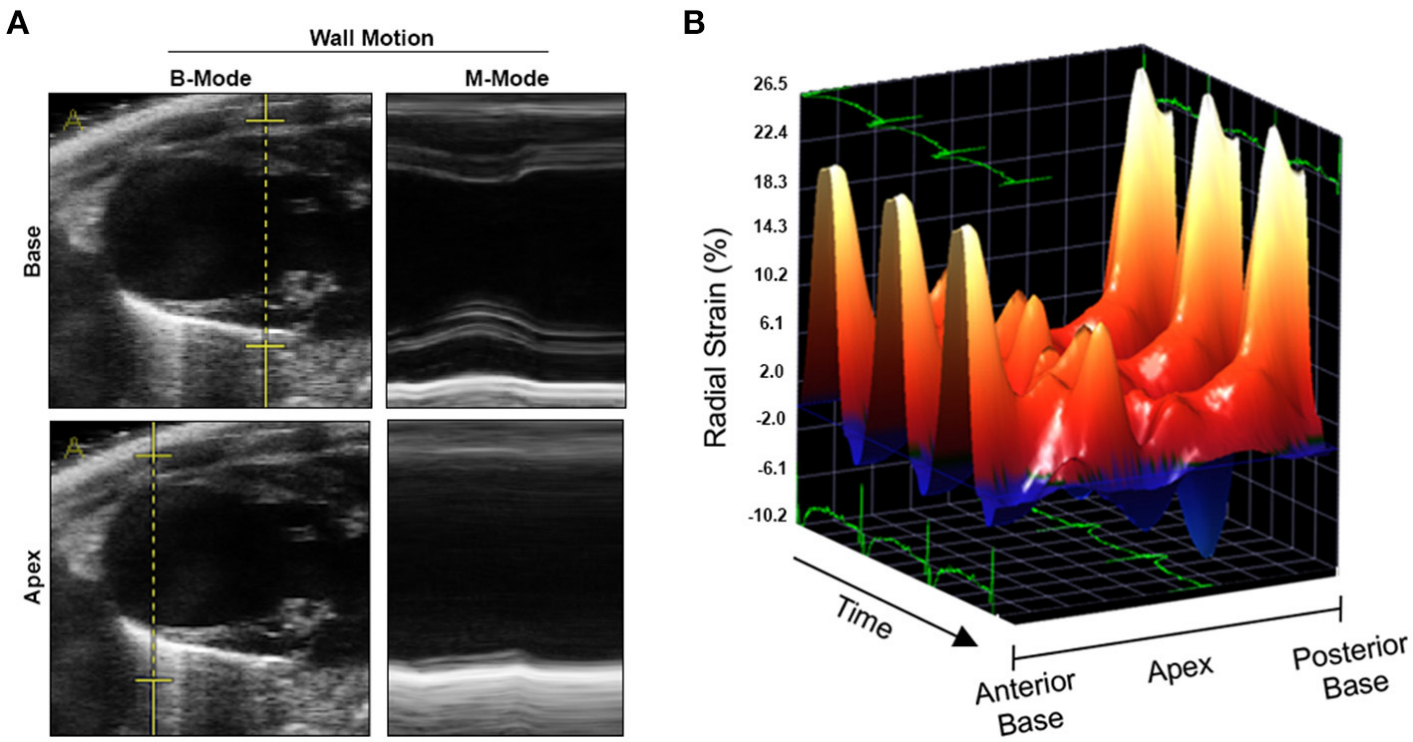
Wall motion analyses by conventional and speckle-tracking echocardiography. (A) Reconstructed M-modes at basal (upper panel) and apical level (lower panel) indicating apical akinesia. (B) Three-dimensional reconstruction of radial strain during three cardiac cycles. Three-dimensional Cartesian coordinate system mapping radial strain, time/cardiac cycles, and myocardial segments derived from the cardiac long axis (from anterior base over apex to posterior base). Radial strain in midmyocardial and apical segments (both anterior and posterior) was markedly lower as compared to basal segments.
